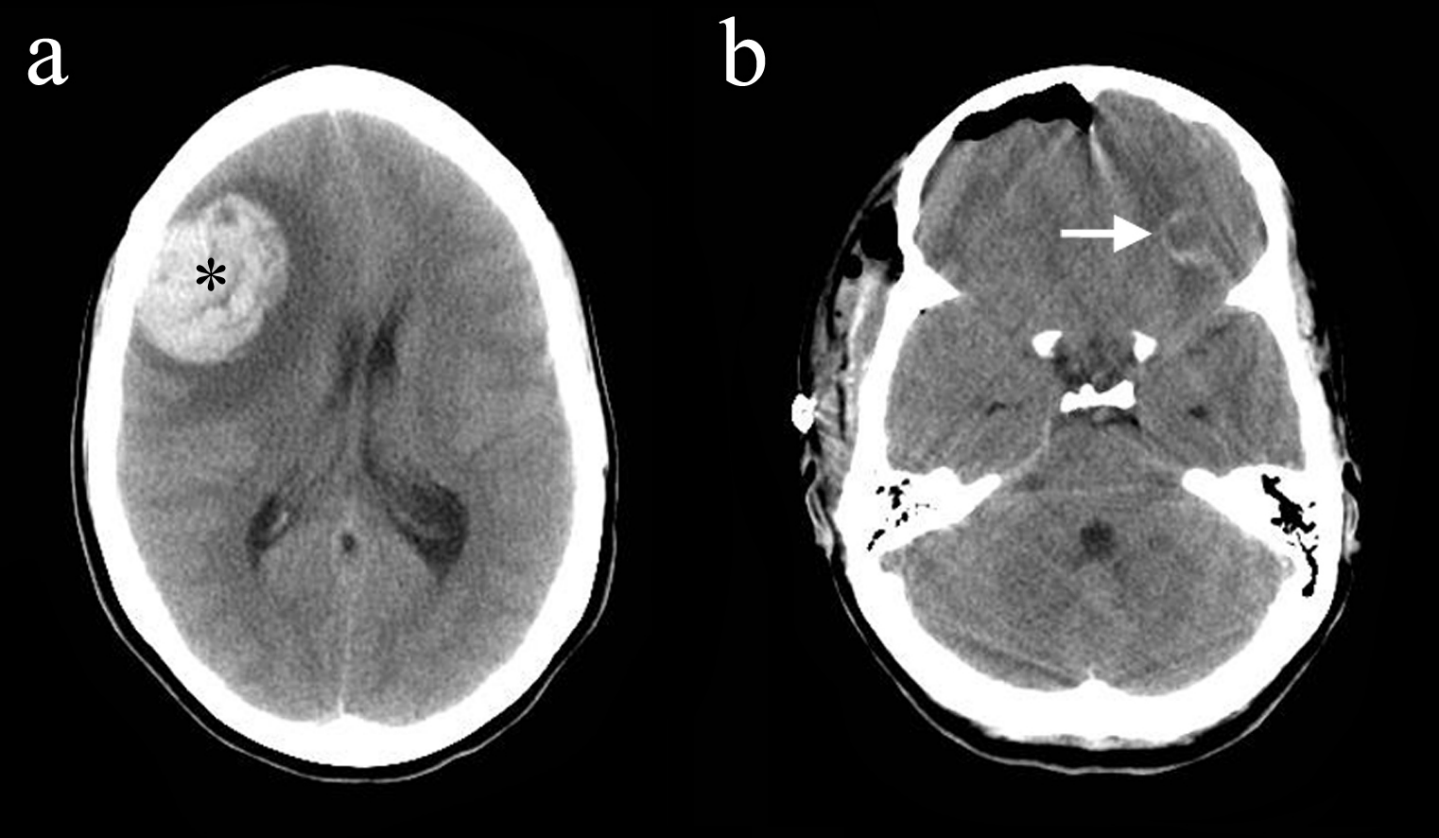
a Axial image from the initial unenhanced CT brain study showing a large heterogeneous high density lesion in the right frontal brain parenchyma consistent with an acute hematoma (asterisk) with the surrounding low density of vasogenic edema causing midline shift to the left. The presence of several smaller ring lesions (see b) combined with the clinical history led the reporting neuroradiologist to raise the possibility of intracranial mycotic aneurysms as well as cerebral abscesses. b Unenhanced axial CT brain image post-craniotomy and hematoma evacuation (note the pneumocephalus). This demonstrates one of the ring lesions in the inferior left frontal region (arrow) that was noted in the initial study. This showed rim enhancement with intravenous contrast administration (not shown), in keeping with the diagnosis of a cerebral abscess.
